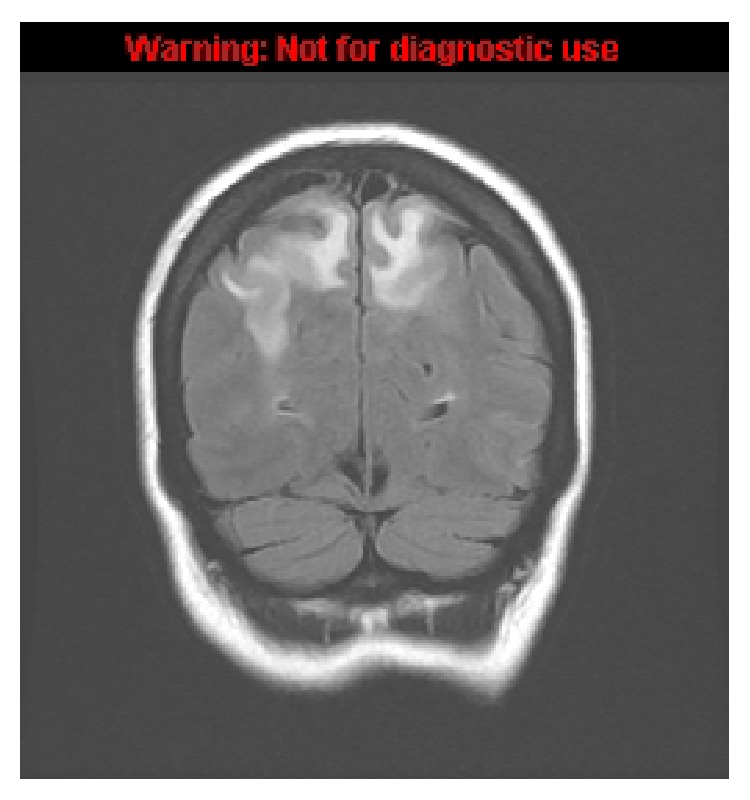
Coronal FLAIR image demonstrating hyperintensity in the posterior occipital white matter extending up to the vertex with mild local sulcal effacement consistent with posterior reversible encephalopathy syndrome (PRES).
